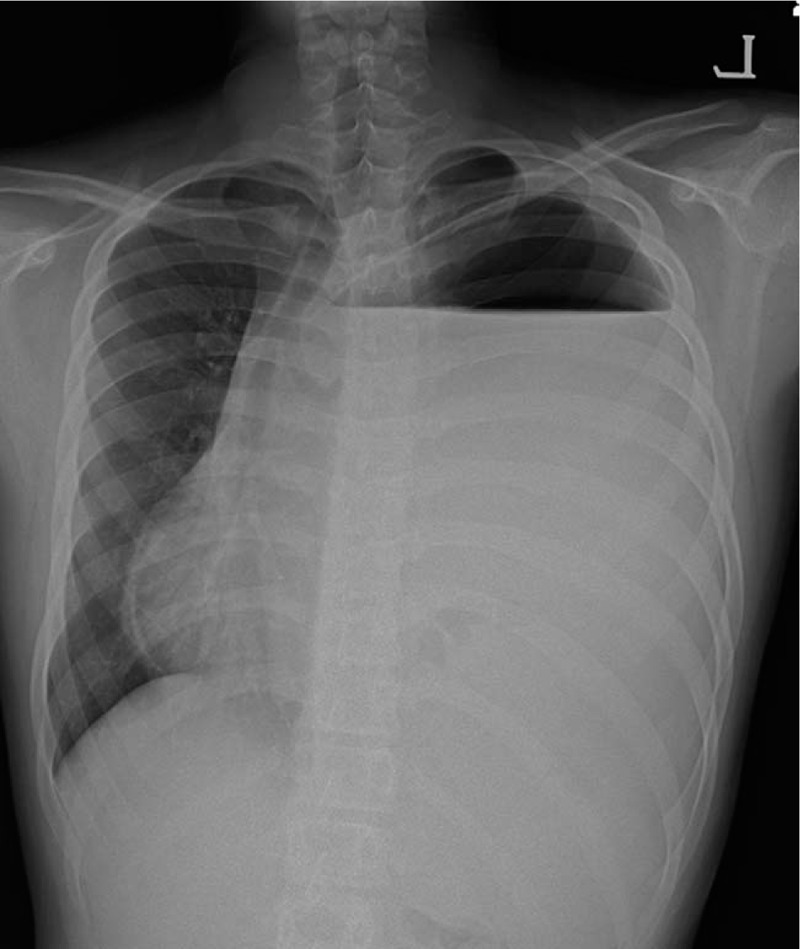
Initial chest x-ray shows an opacified hemithorax with contralateral shift of the mediastinum, which mimics pleural effusion.
